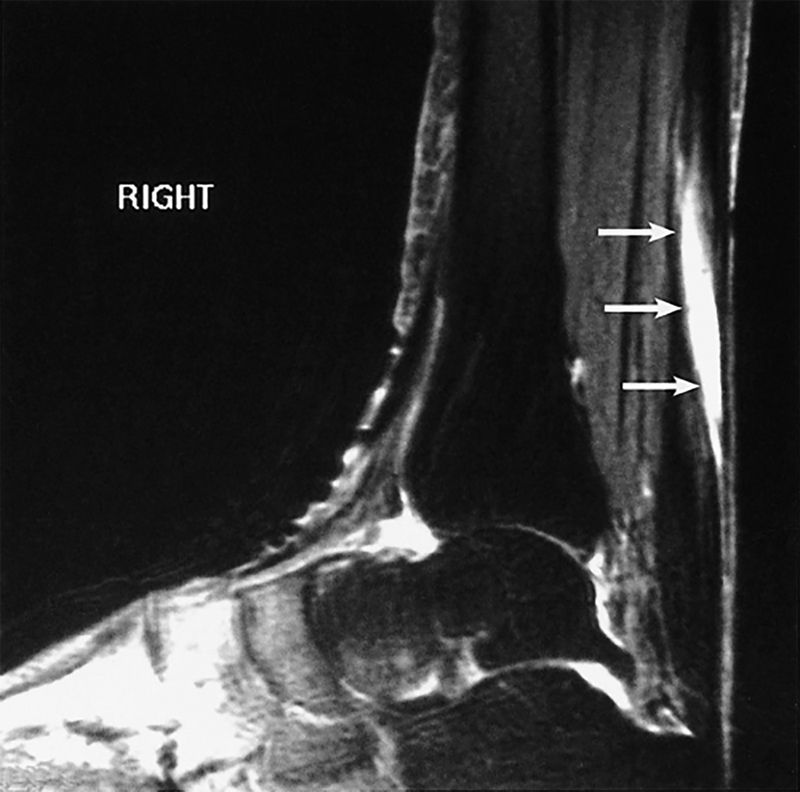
Magnetic resonance imaging of fluoroquinolone-induced Achilles tendon rupture. Sagittal T2-weighted fat-saturated image shows a high-grade tear at the musculotendinous junction. (Reproduced with permission from Yu C and Giuffre B.
